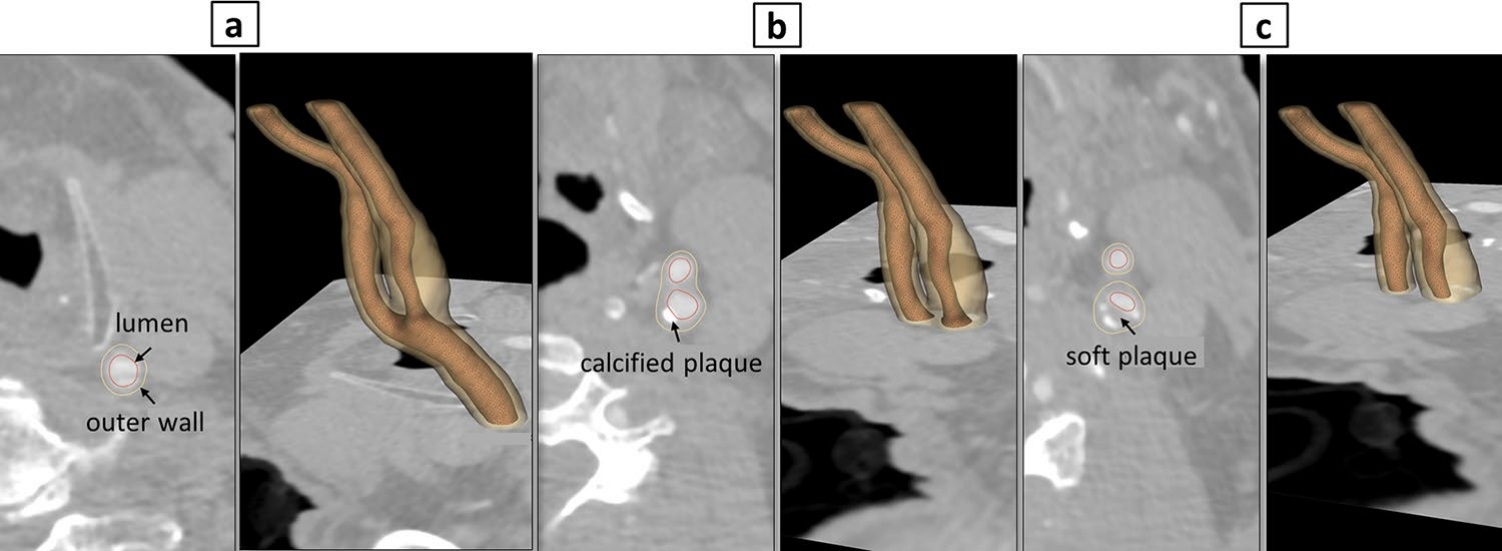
CTA slices with 3D projection of reconstructed carotid artery bifurcation: lumen and outer wall at CCA (a), bright spot showing calcified plaque at ICAs region (b), soft plaque seen between the calcified plaque and lumen (c).
